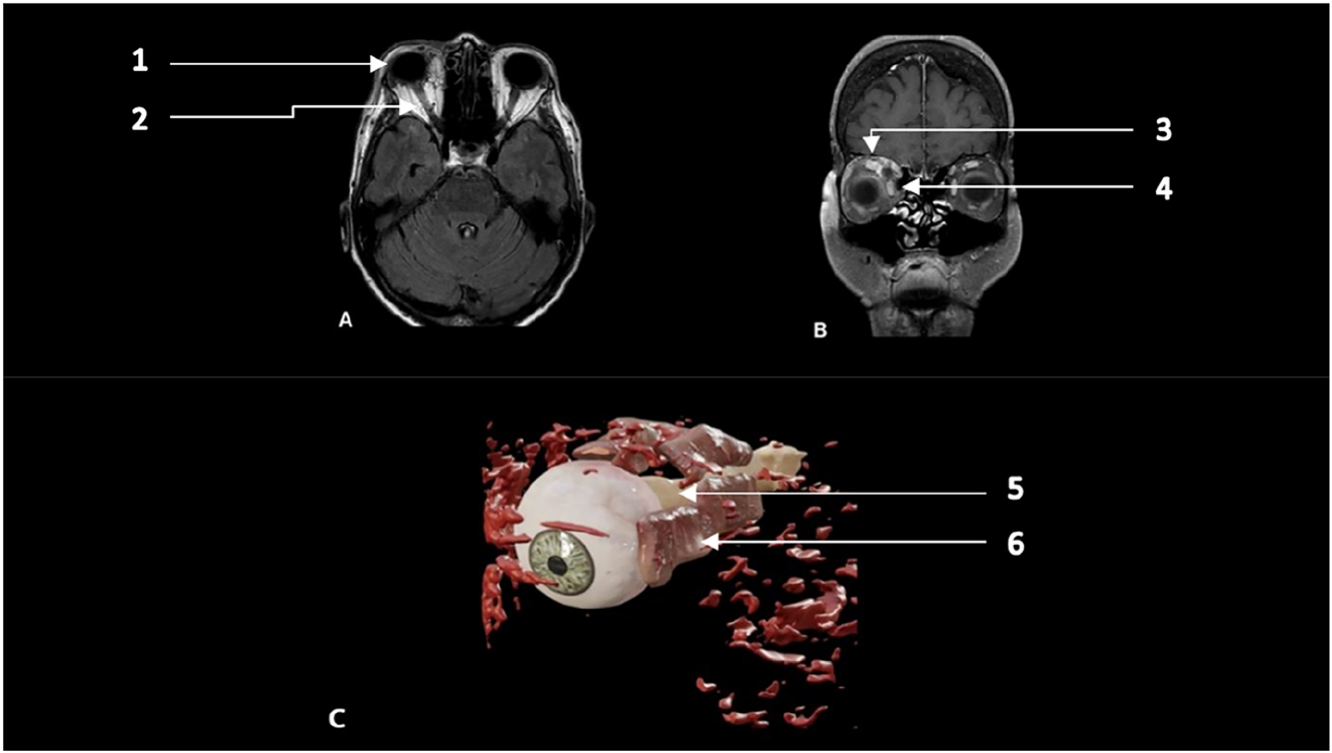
Postoperative MRI and Volume Reconstruction. (A) Axial and (B) coronal T1-weighted image with contrast on the level of orbit. (1) Globe, (2) optic nerve, (3) *M. rectus* superior, (4) *M. rectus* medialis. (C) Volume reconstruction of eyeball and orbital muscles. (5) optic nerve, (6) *M. rectus* medialis.
